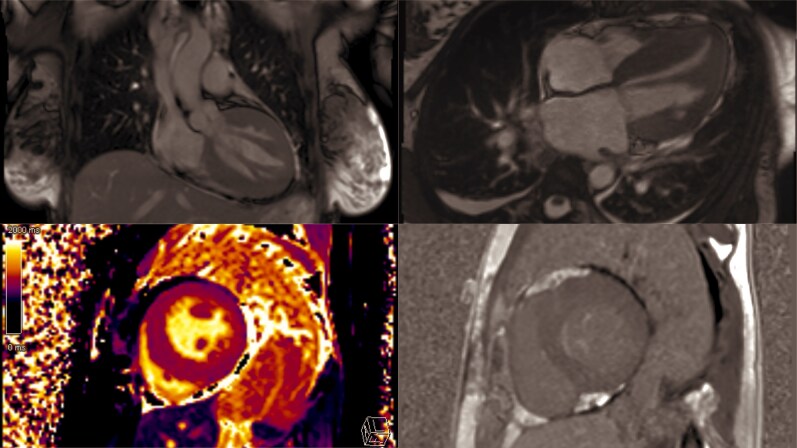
Multiplanar cardiac MRI assessment demonstrating left ventricular morphology, function, and tissue characteristics.
